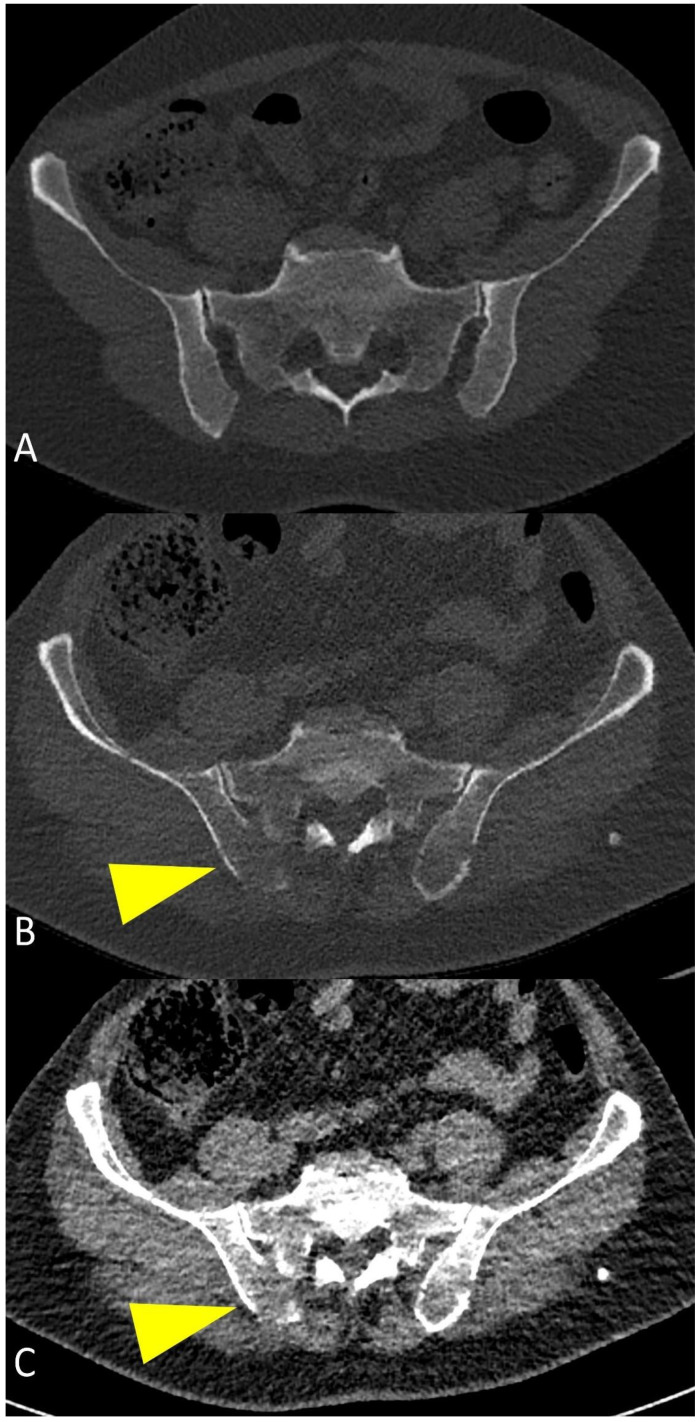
(A) Axial CT images showing normal appearance of bone and bone marrow in an axial skeleton with dense trabeculae. (B,C) myeloma-related osteolysis appearing as focal destructive lesions of the trabecular bone with cortical interruption and without a sclerotic border (yellow arrowheads).
